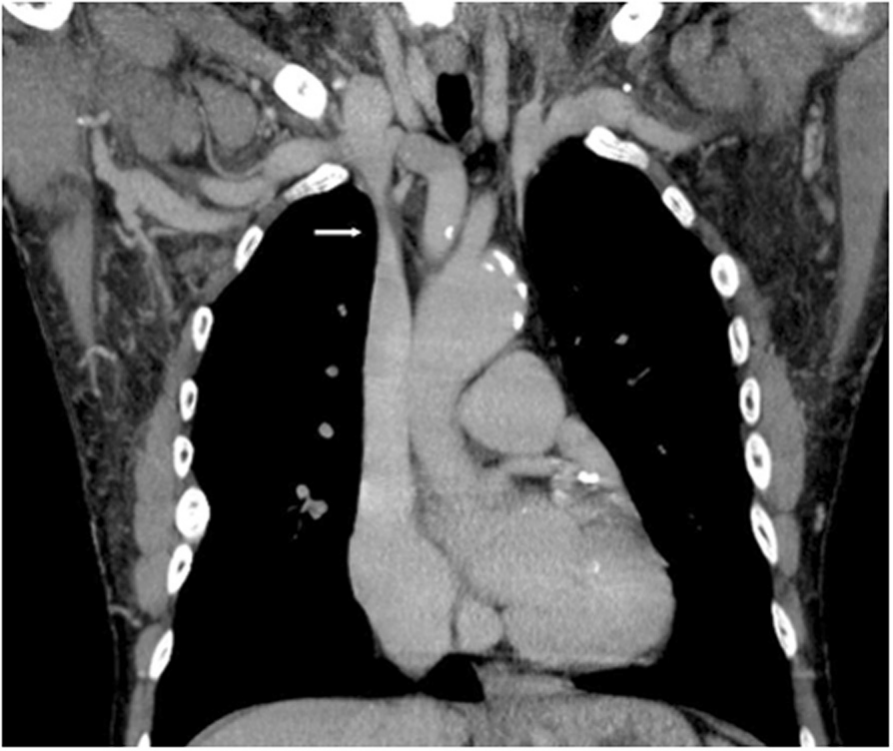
Computed tomography venography, coronal view of the upper chest. The high grade stenosis of the brachiocephalic vein is marked by an arrow.
